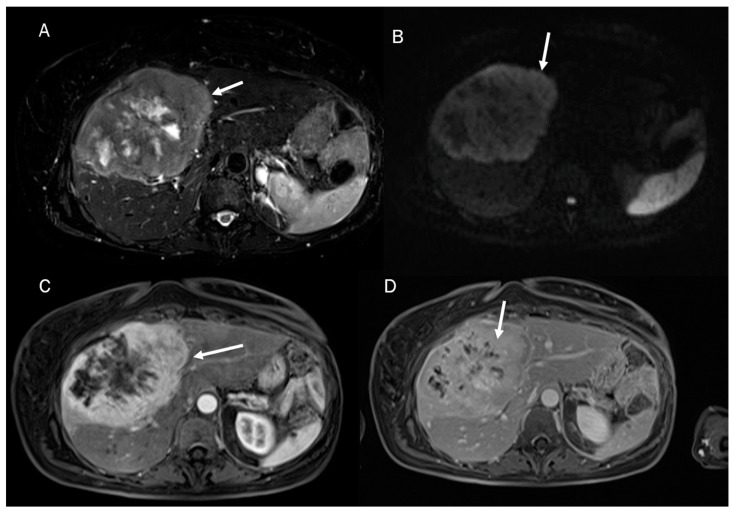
Combined hepatocellular cholangiocarcinoma MRI assessment: the lesion (arrow) shown in T2-W sequence (A); targetoid appearance, with restricted diffusion in b800 s/mm^2^ (B); and progressive contrast enhancement during contrast study (arterial phase (C) and portal phase (D)).
